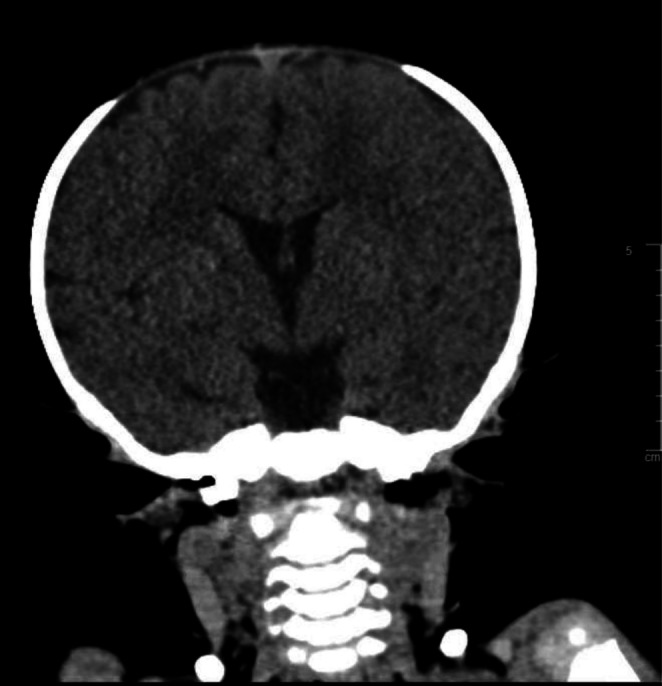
Non‐contrast CT brain at 15 months of age showing preserved brain parenchyma. There was widening of anterior fontanelle and sagittal sutures. The coronal sutures and particularly the temporoparietal sutures don't seem to be accurately open.
